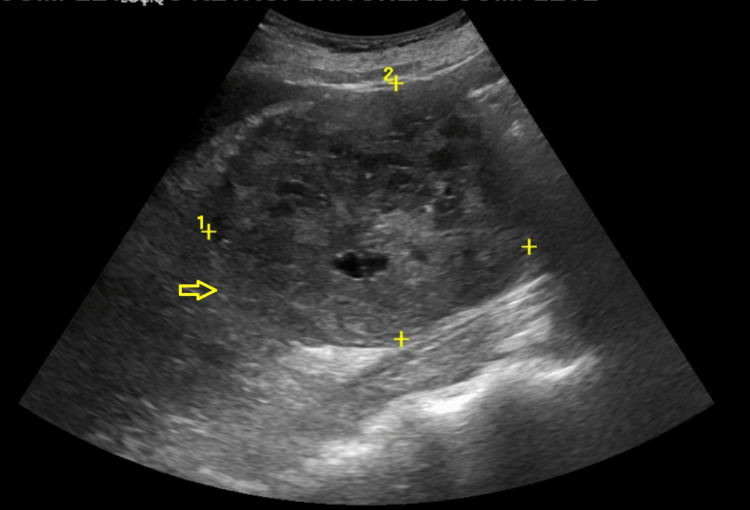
A 10.2 x 8.1 cm heterogeneous partially cystic and partially solid mass (arrow) arising from the lower pole of the left kidney seen on renal ultrasound - suggestive of RCC
